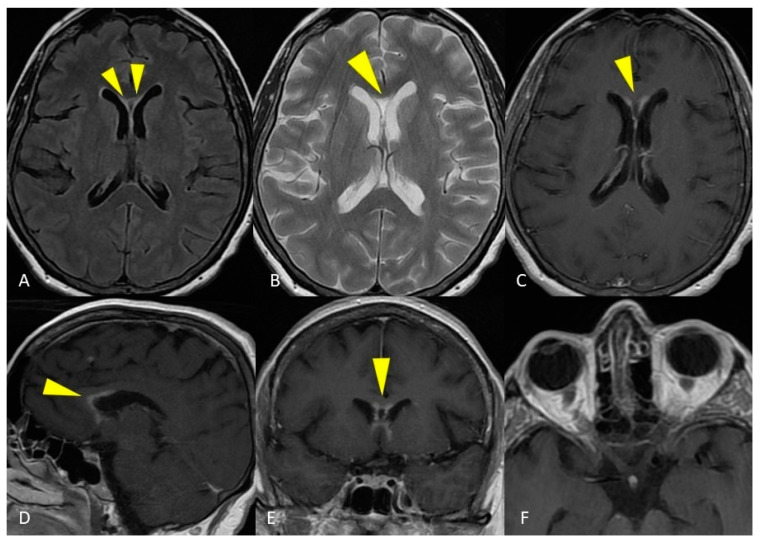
MRI of the brain. Axial T2-weighted and FLAIR images show curve hyperintense lesions affecting the ependymal surface of frontal horns of the lateral ventricles and corpus callosum just near the genu, with a symmetrical pattern (A,B). These sub-ependymal lesions show homogeneous enhancement on the T1-weighted post-gadolinium image (C–E). No optic nerve enhancement on T1-weighted post-gadolinium images was present (F).
